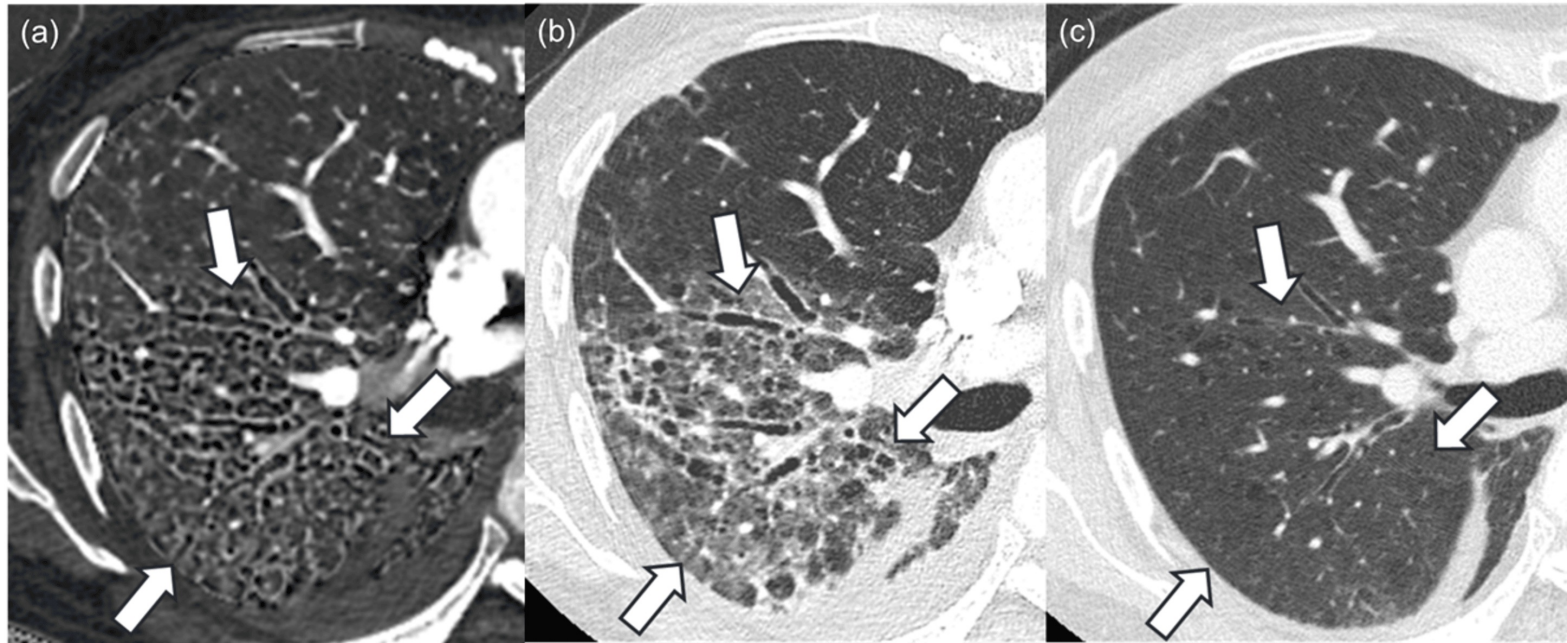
Images of a patient diagnosed with antisynthetase syndrome. The iodine map (a) and monochromatic image (b) of a 72-year-old female patient diagnosed with antisynthetase syndrome. Bronchiectasis and airspace enlargement, which are presented as black tiny structures in the region surrounded by white arrows, are more prominent in the iodine map compared to the monochromatic image, and readers 1, 2, and 3 rated 0 (same degree), 1 (iodine map is prominent), and 1 in side-by-side qualitative evaluation, respectively. Bronchiectasis and airspace enlargement were ameliorated in the evaluated region in the follow-up CT examination (c), which was performed after 100 days. Window level/width for iodine map (a) and CT images (b, c) were 15/110 mgI/ml and –600/1600 Hounsfield unit, respectively.
